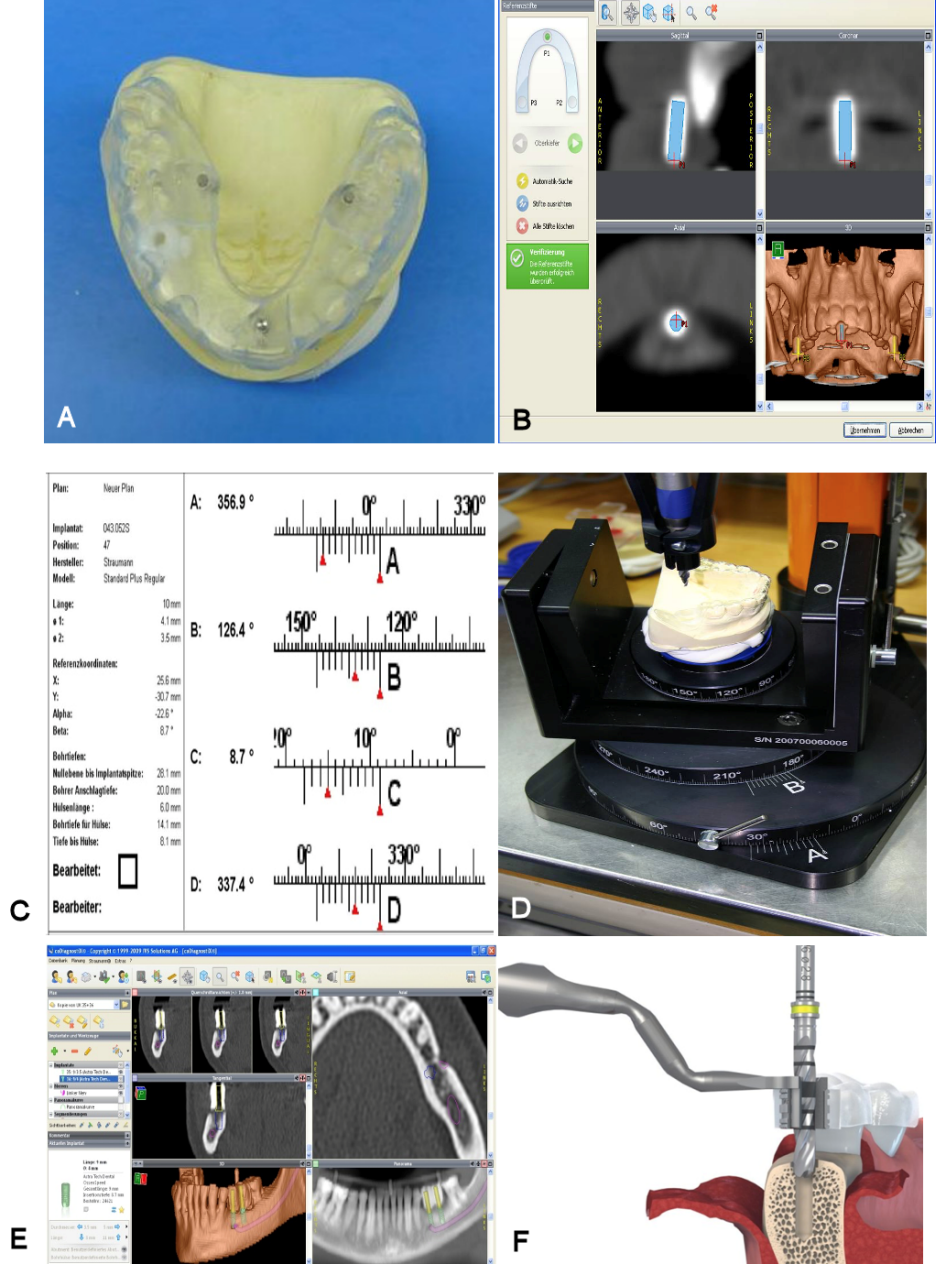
Technical way to manufacture the surgical drilling template, applying the prosthetically-guided planning concept by using digital planning software. A: Radiographic template with barium sulfate tooth 014 and the radiopaque markers. B: The radiopaque markers in the software display. The correction of the automatic detection is done manually if necessary. C: Planning data of an implant regio 047. On the left in the upper the selected implant system with the corresponding length and diameter can be seen. On the right and bottom left, the data important for fabricating the surgical guide are displayed. D: After setting the drilling table in 4 planes (A, B, C, D) according to the planning data, the drill hole is made in the drilling template. The metal die is then glued in place. E: Planning interface of the software. The implants were selected according to manufacturer, type, and diameter and placed in the jaw in the ideal prosthetic position shown by the X-ray template. The position data were sent to the laboratory for fabrication of the surgical guide. If there was no bone available, the bone augmentation was planned or, as in the present example, inserted paranervally to avoid costly vertical augmentations. F: Representation of the Straumann Guide System. Similar to the Steco system, however, here the drill sleeves are located on a guide tray to simplify handling. © Institut Straumann AG
